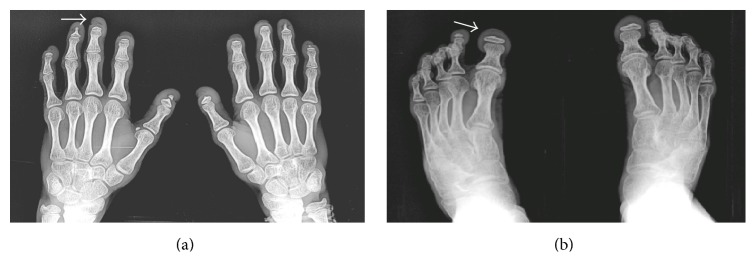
Anteroposterior radiograph of both hands (a) and foot (b) showed resorption of terminal phalanges with preservation of soft tissue (white arrow).
